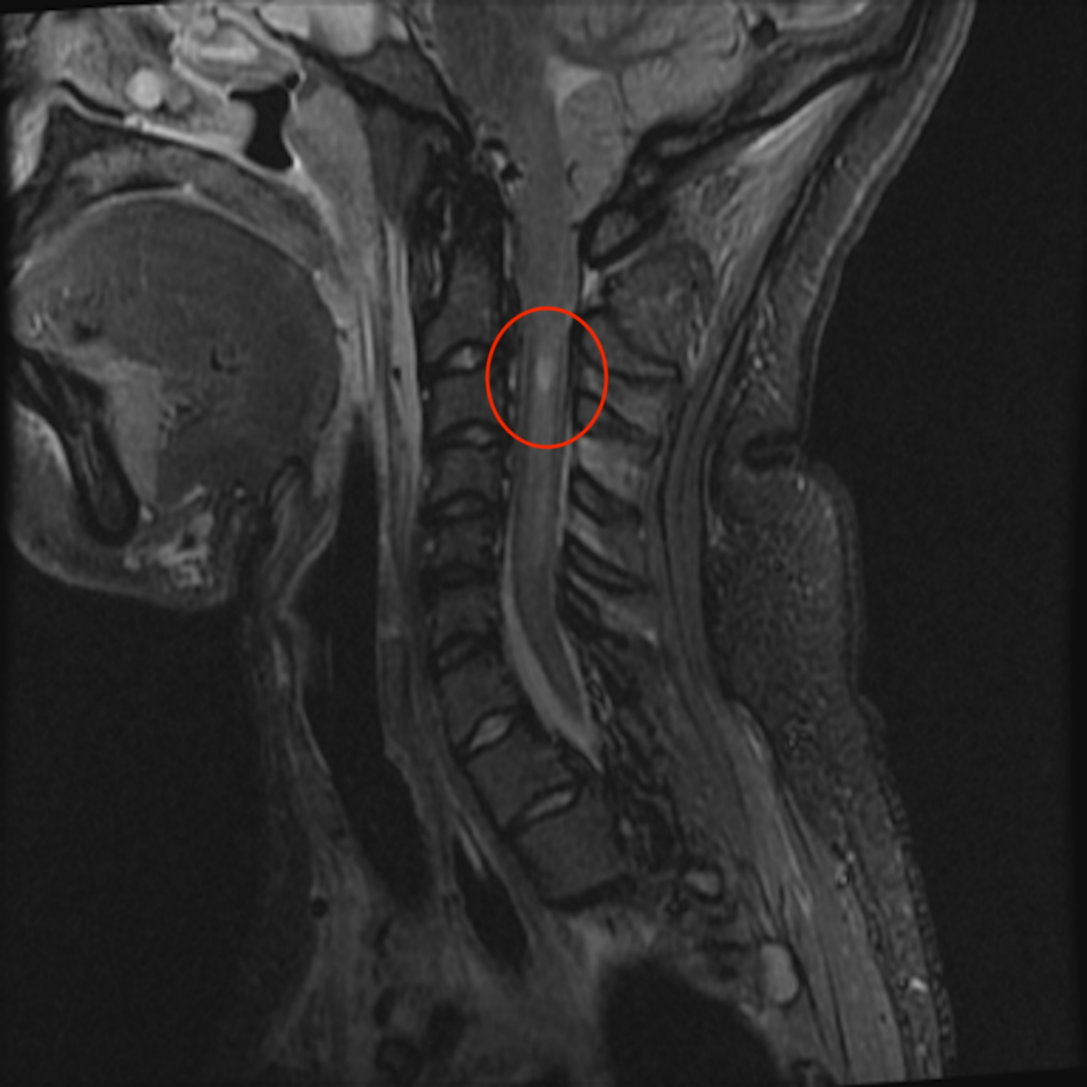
Magnetic resonance imaging (MRI). MRI brain showing resolved presyrinx along with cerebrospinal fluid (CSF) in premedullary region.
